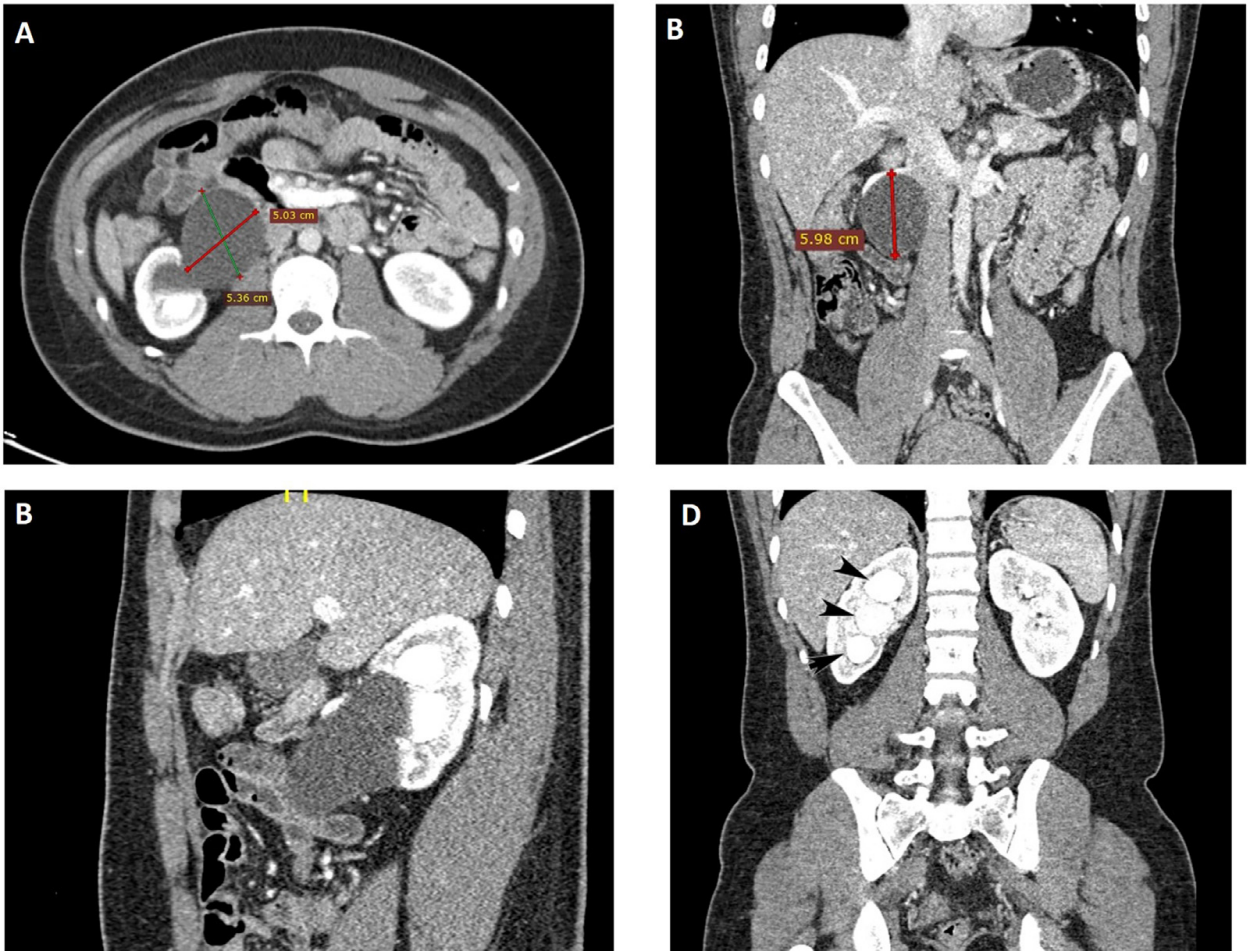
Contrast CT scan demonstrated the PPC with hypodense lesion (A) Transverse view with a dimension of the cyst measuring 5.03 cm (red line) x 5.36 cm (green line), (B) Coronal view with dimension of the cyst measuring 5.98 cm (red line), (C) sagittal view, (D) coronal view Right calyceal dilatation indicating obstruction.
